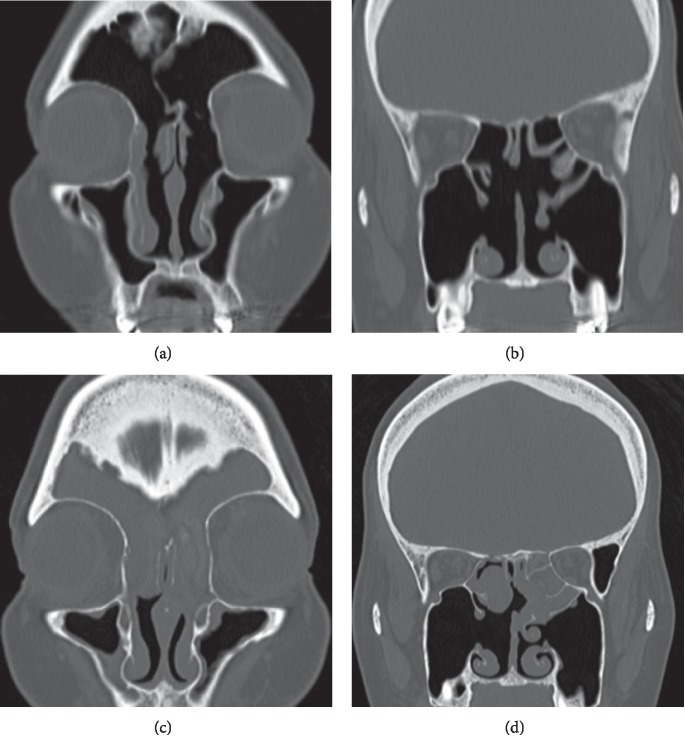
Case 1 recurrence. (a, b) Computed tomography (CT) image of the paranasal cavity in Case 1 after the first surgery. Pathways from the nasal cavity to each paranasal cavity are cleared. (c, d) CT image of the paranasal cavity in Case 1 after disease recurrence. Contrasts are evident in the ethmoid and frontal sinuses on both sides.
